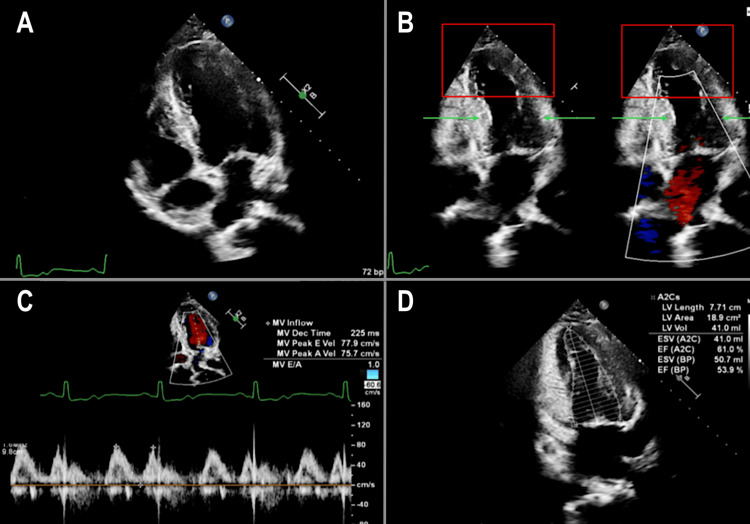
(A) Apical four-chamber view at end diastole. (B) Apical four-chamber view at end systole. Red boxes showed the hypokinesis of the left ventricular apical and apical inferior septal wall. (C) Decreased E to A ratio of the left ventricle. (D) The left ventricular ejection fraction is estimated to be 53% by the Simpson's summation of disks method.
